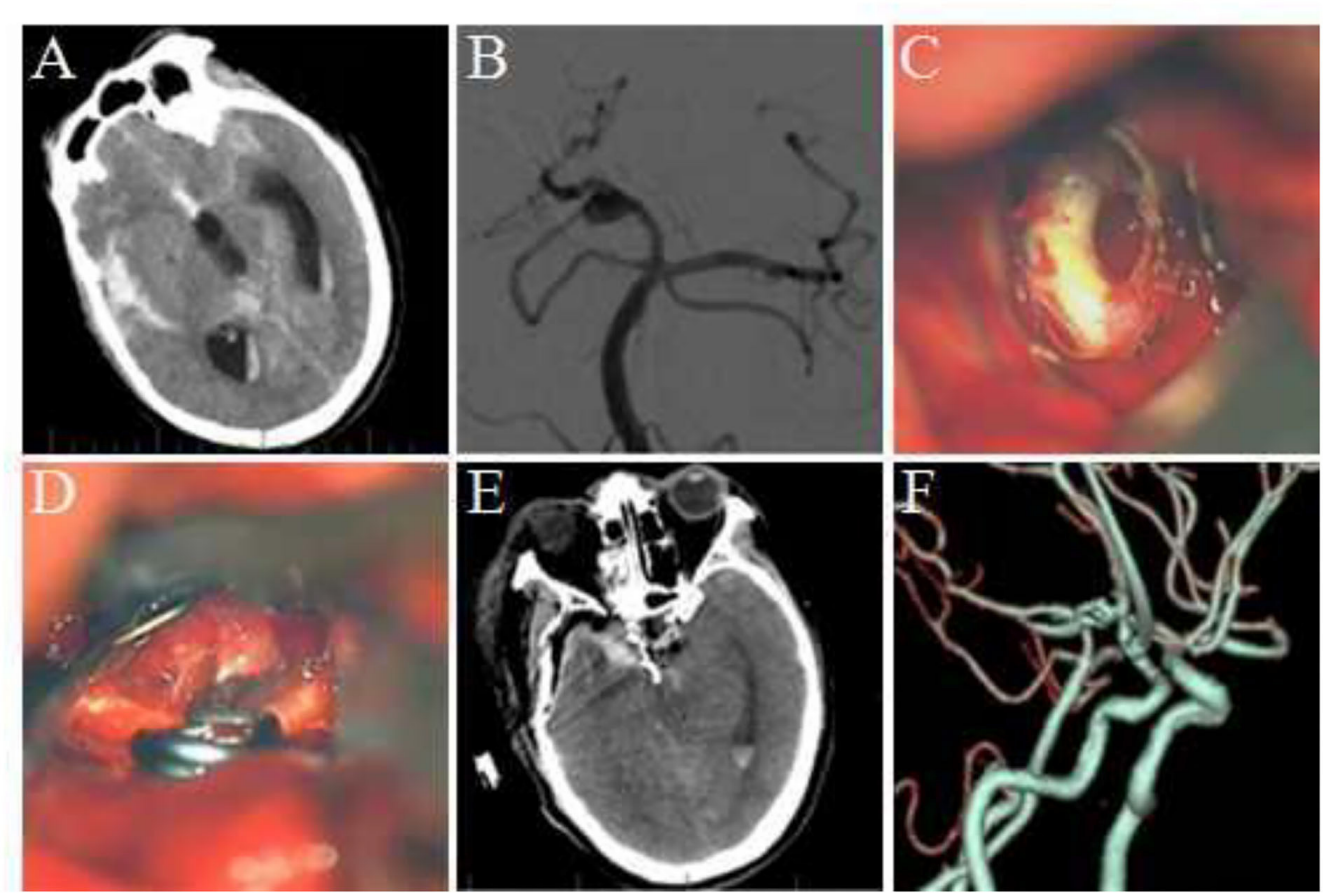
Clipping of the aneurysm at the P1–P2 bifurcation of the posterior cerebral artery *via* the Dolenc approach. (A) Preoperative head CT revealed subarachnoid hemorrhage. (B) DSA revealed the tumor at the P1–P2 bifurcation of the right posterior cerebral artery. (C) The aneurysm was exposed during the surgery; the wall of the apical aneurysm was thin, and adhesion was observed between the aneurysm and temporal lobe. (D) The aneurysm was clipped parallel to the parent artery during the surgery. (E) Re-examination using head CT within 12 h after surgery. (F) Postoperative CTA revealed no development of aneurysm and no vascular damage.
